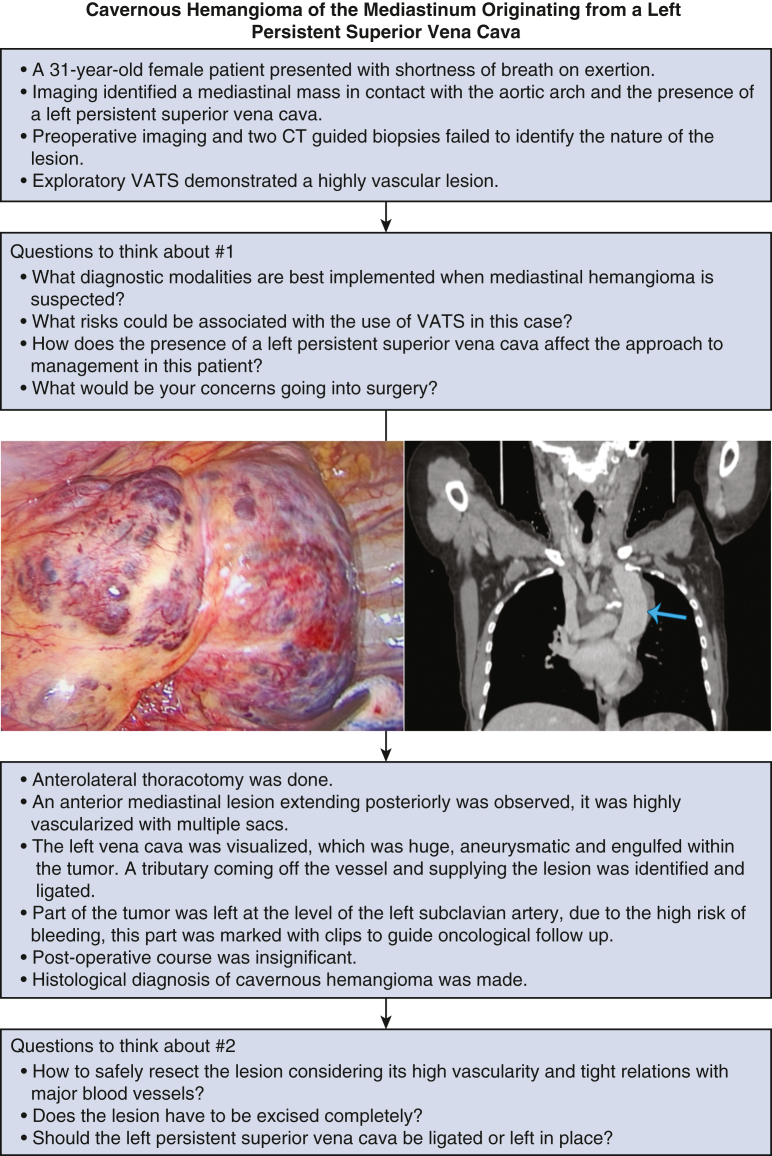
Questions and interesting points in the diagnosis and management of patients with rare mediastinal hemangiomas. *Blue arrow* indicates the dilated left superior vena cava preoperatively. *CT*, Computed tomography; *VATS*, video-assisted thoracoscopic surgery.
